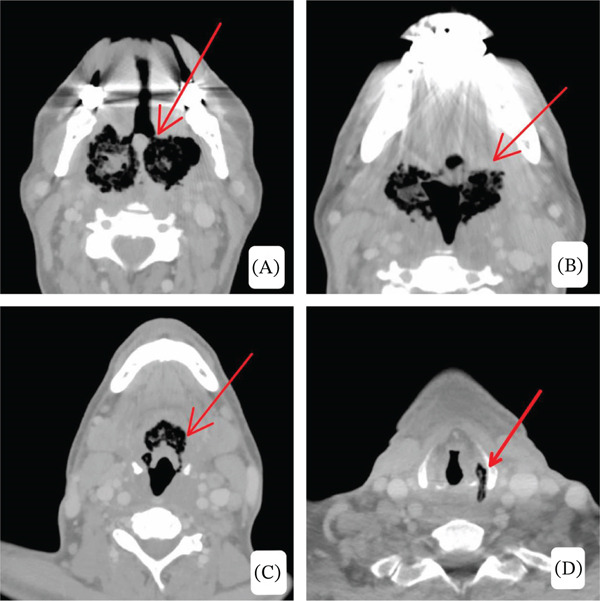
(A–D) Axial contrast‐enhanced CT images at multiple levels demonstrate central and submucosal gas locules within the palatine tonsillar parenchyma, causing diffuse almost symmetrical bilateral tonsillar enlargement and surrounding inflammatory changes, with extension into the adjacent parapharyngeal space. (C) At the periepiglottic level, there is circumferential inflammatory involvement with gas locules, resulting in significant airway narrowing. (D) At more inferior levels, there is involvement of a left‐sided laryngocele, which also contains gas and inflammatory changes, reflecting inferior extension of the same emphysematous infectious process.
